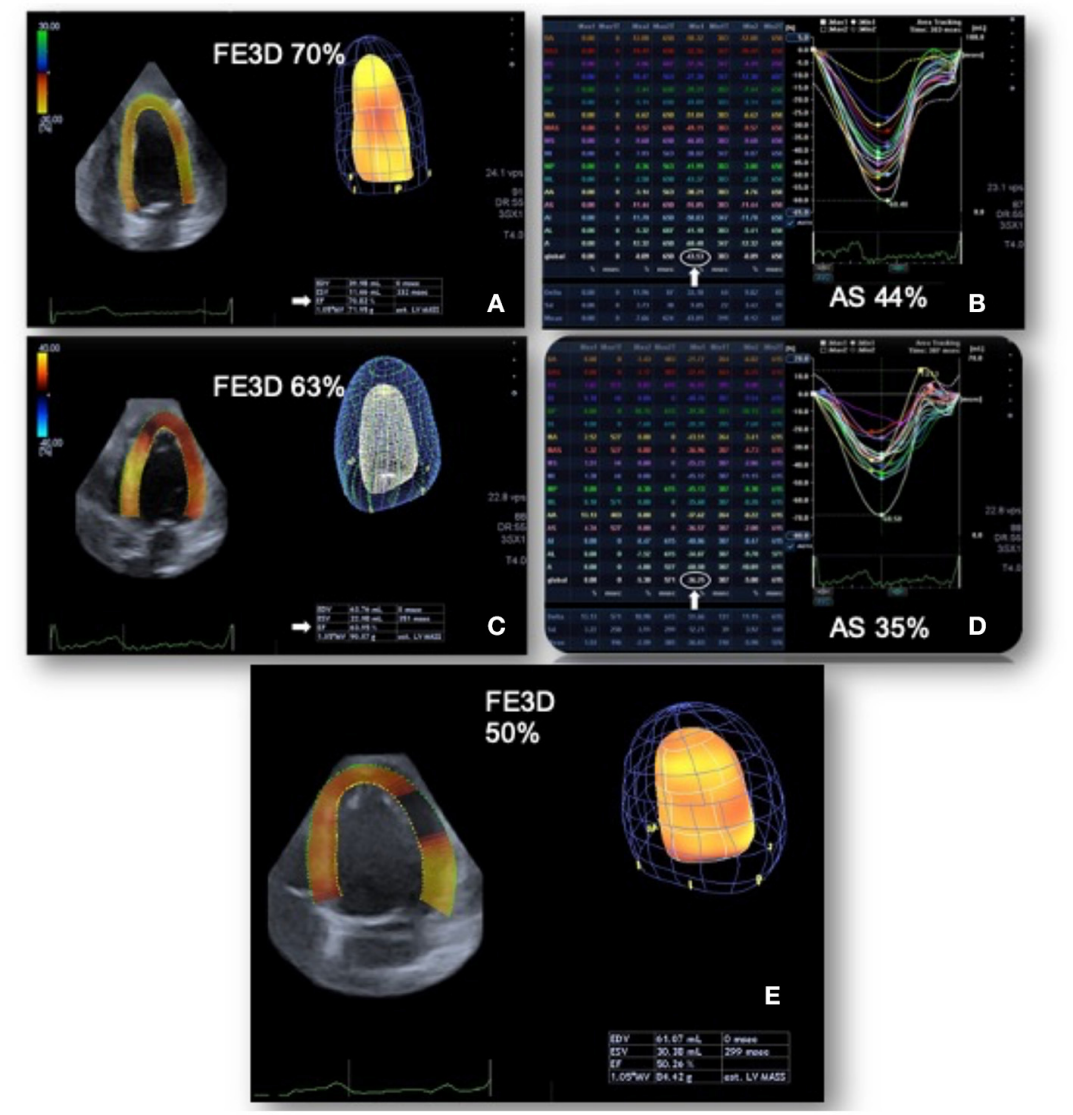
Changes in global area strain derived from the three-dimensional speckle tracking echocardiography. Patient with breast cancer undergoing chemotherapy with doxorubicin. In the baseline evaluation, pre-chemotherapy, left ventricular ejection fraction (LVEF): 0.70 and 3DGAS: −43.5% (A,B). After a low cumulative dose of doxorubicin (120 mg/m^2^), LVEF: 0.63 was observed (with reduction, but still preserved) and a significant decrease in three-dimensional global area strain (3DGAS) to −36.2% (C,D). Six months after the end of chemotherapy with doxorubicin (total cumulative dose of 240 mg/m^2^), the LVEF dropped to 0.50 (E). GAS, global area strain; LVEF, Left ventricle ejection fraction.
